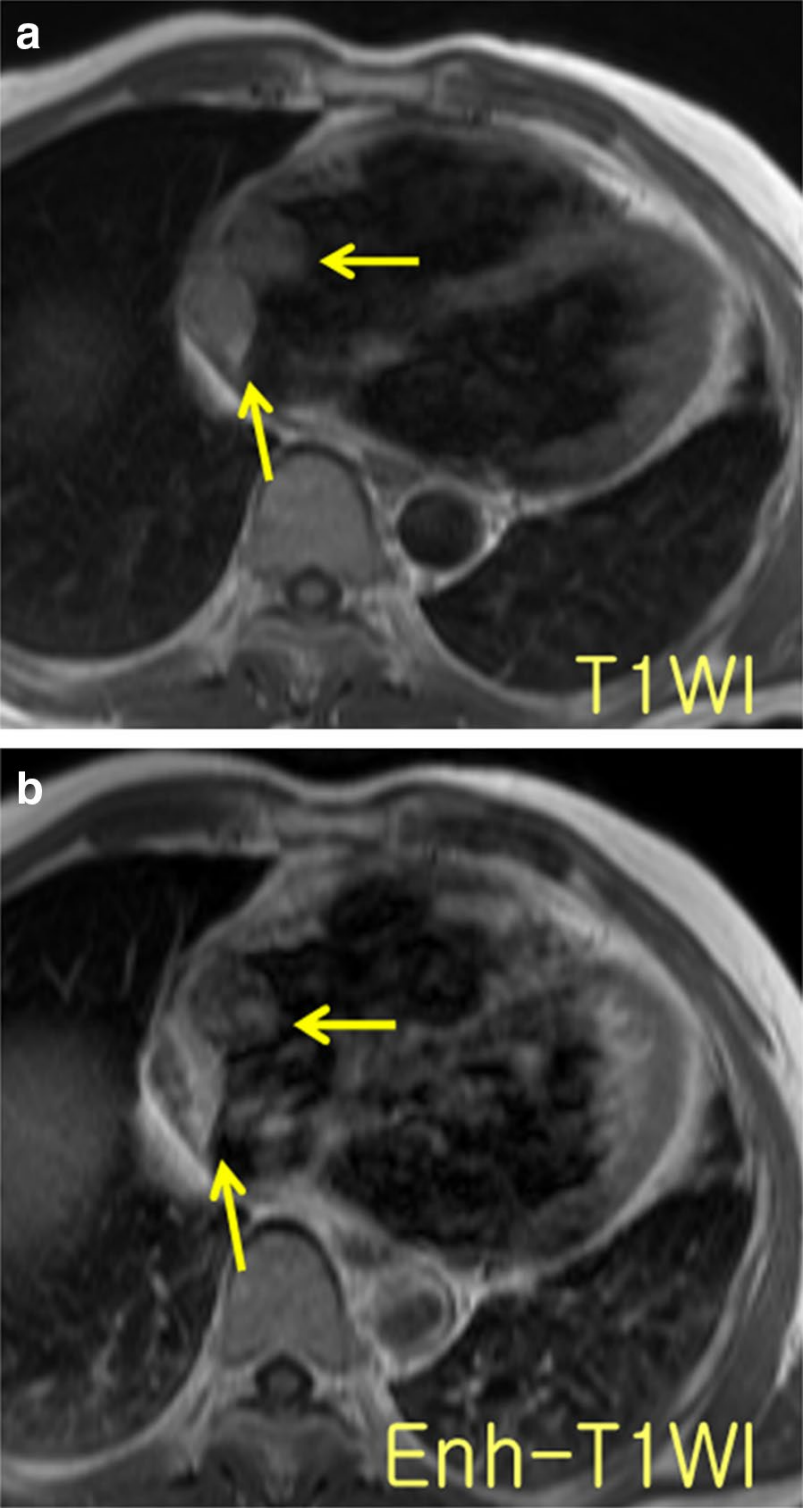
a Double inversion-recovery T1-weighted image demonstrates a relatively isointense lobulated mass in the free wall of right atrium. b Gadolinium-enhanced T1-weighted image shows heterogeneous enhancement of the mass. Within the mass, some portions had no enhancement, representing intra-tumoral thrombosis or necrosis
